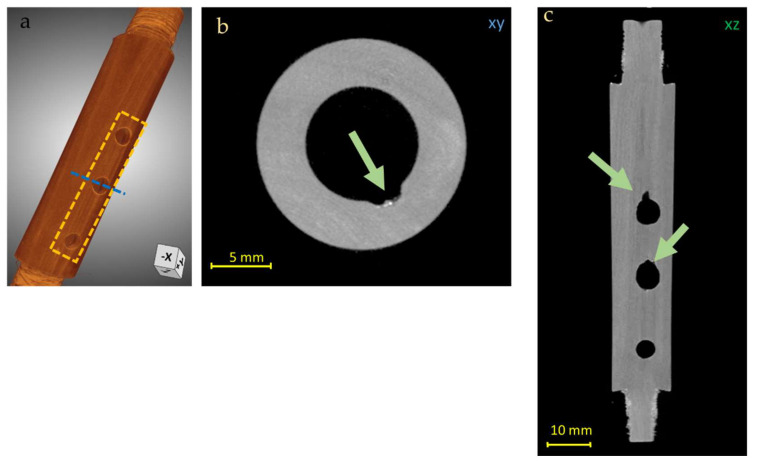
3D render of the Piccolo Flute body (a) and CT horizontal (b) and vertical (c) slices of the Piccolo Flute body, in which imperfections in the holes are observed (green arrows in (b,c), yellow square in (a)). Blue line in (a) indicates the high of the XY slice (b).
